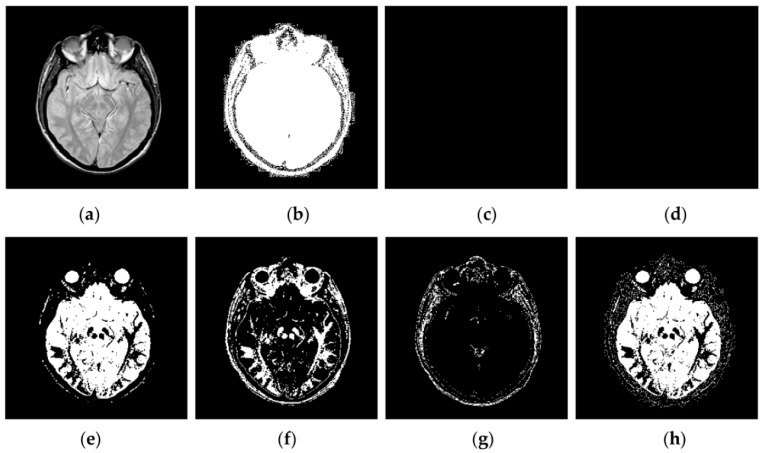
Temporal series of pulse outputs generated by the SCM operating on magnetic resonance (MR) image: (a) MR image; and (b–h) the binary pulse images from the first to the seventh iteration, respectively.
